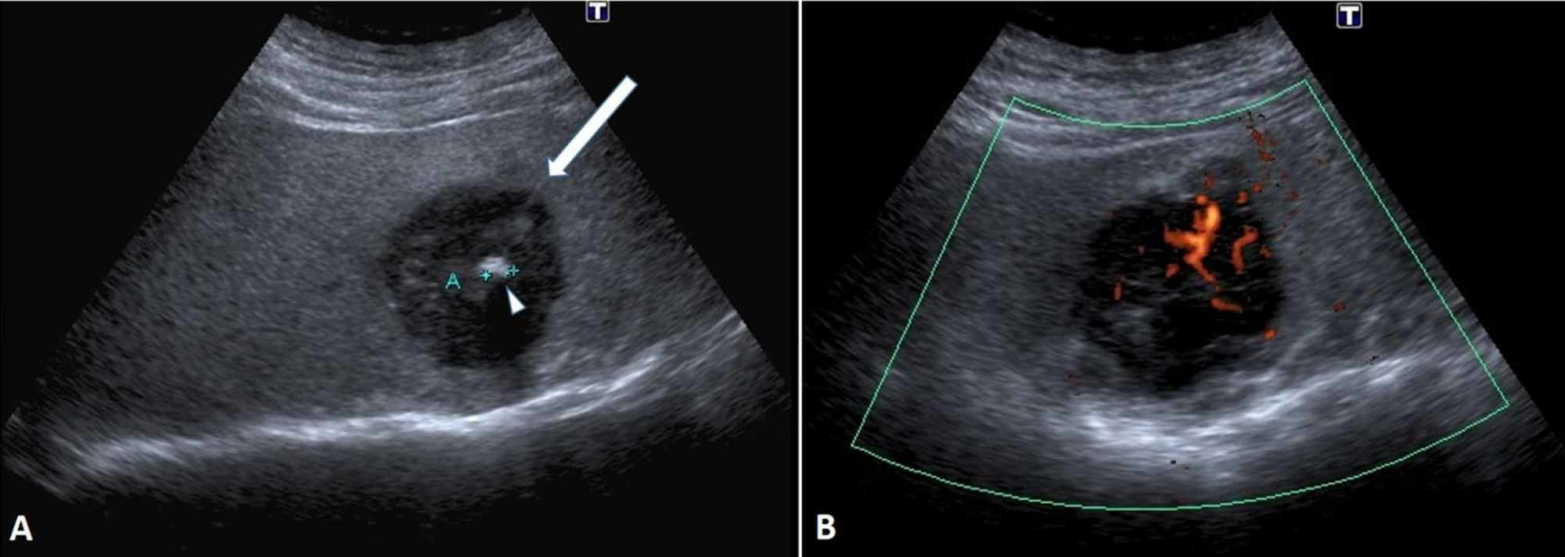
Liver mass. (A) US exam shows diffuse hyperechogenic (fatty) liver parenchyma and moderately hypoechogenic mass (arrow) with central hyperechogenic core (arrowhead). At the power Doppler (B) a central artery with spoke-wheel (centrifugal) flow is demonstrated. US, ultrasound
